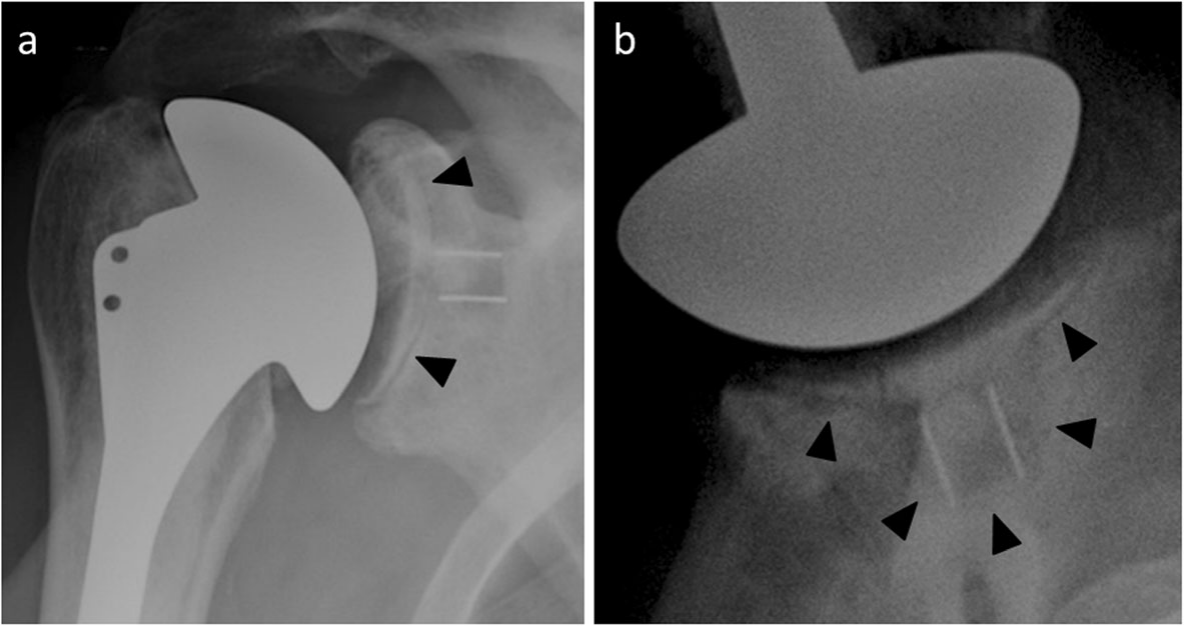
Anteroposterior (a) and axillary (b) radiographs in a 66-year-old man with shoulder pain show a complete radiolucent line around the glenoid component revealing glenoid loosening
